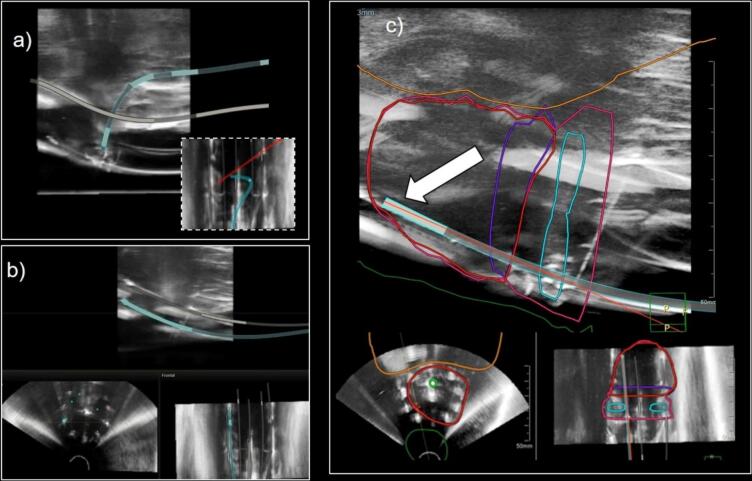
EM tracking in an MRI-US fusion scenario. US-to-EM reference frames calibration allow the EM navigation on the US. (a) The stylet is used to digitally reconstruct the applicator. The stylet path is lost halfway through the reconstruction of the inner channel of the ring, resulting in only half of the ring being reconstructed (as shown in the dotted-frame coronal view). (b) The 6 posterior transvaginal needles are reconstructed with the stylet, while the 3 anterior needles keep their preplanned positions until reconstruction. Those reconstructions are displayed in all three perpendicular views and fit on the US image. (c) The MRI contours are superimposed on the US image. Live tracking of the stylet inserted in the needle is shown by the red line (indicated by the white arrow), allowing to finely adjust the needles depth based on the target contour.
